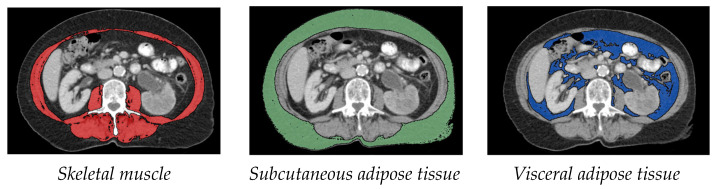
Axial computed tomography images at the third lumbar vertebra region. Ranges of Hounsfield units were used for quantifying different tissues.
